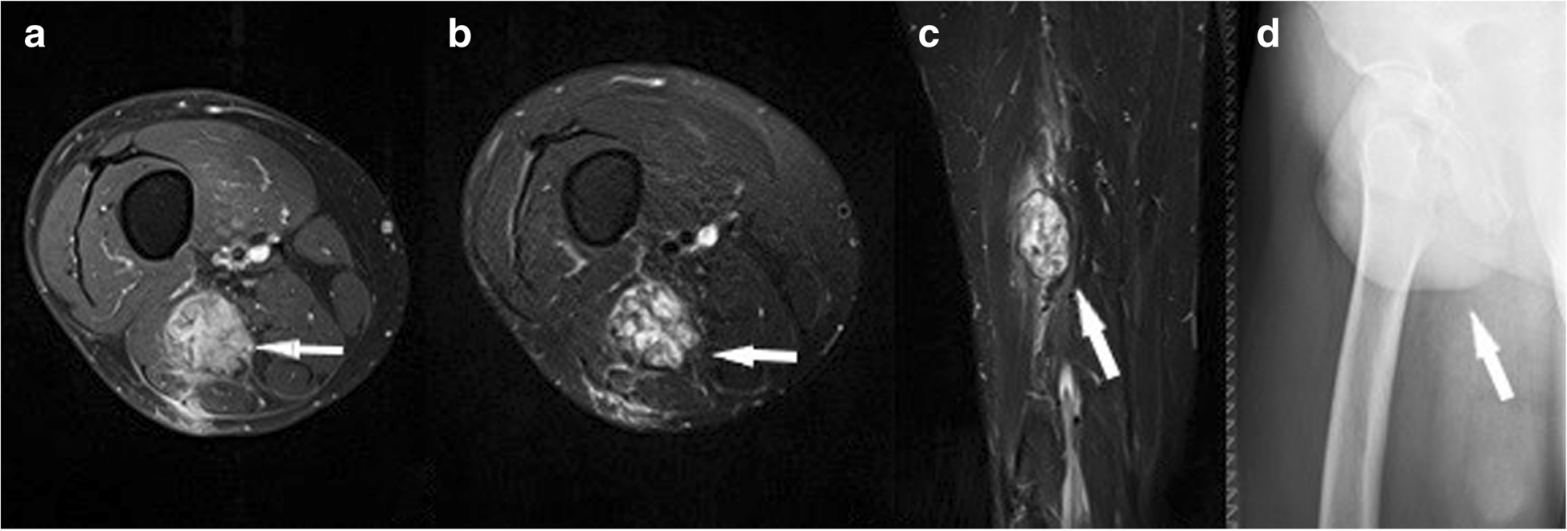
Axial T1fs with contrast medium (a), axial T2fs (b), coronal STIR (c), and standard radiograph (d), depicting myositis ossificans in the hamstrings
